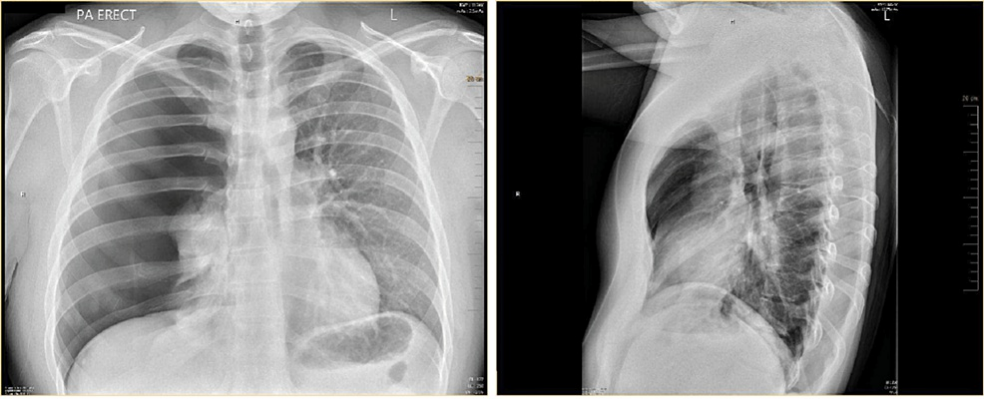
Posteroanterior and lateral chest radiographs upon hospital admission showing large right pneumothorax
